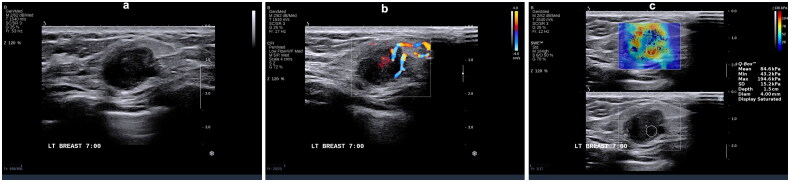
A 30-year-old female with left breast invasive ductal carcinoma grade III (score of 9), tumor size 1.7 × 1.5 × 1.5 cm, triple-negative breast cancer (TNBC). (a) Ultrasonography features: relatively regular morphology, relatively smooth margins, enhanced posterior echo, no calcification. (b) Adler blood flow grading – grade 3. (c) Emax = 194.6 Kpa.
